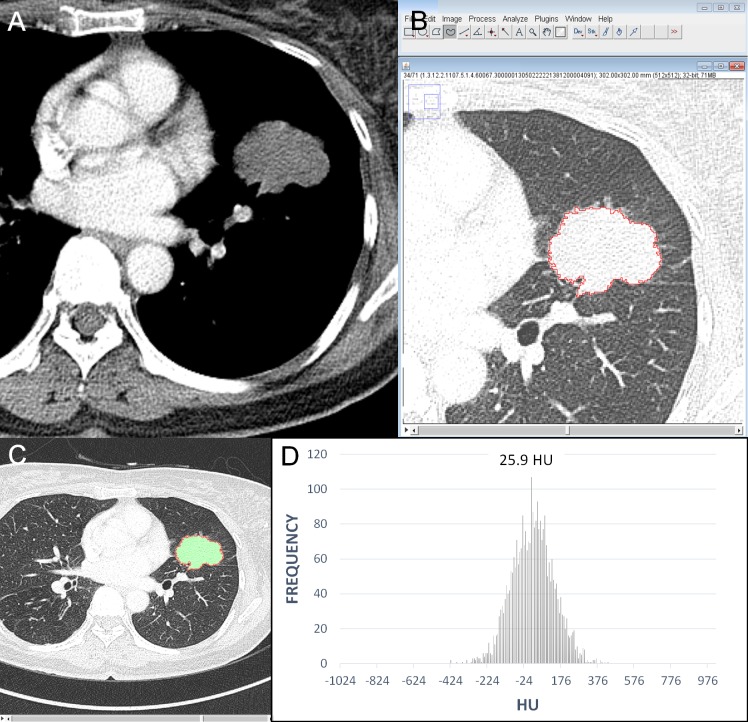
An example of quantitative CT analysis in an adenocarcinoma without recurrence. (A) CT scan and (B) screenshot of quantitative analysis show a 4.6-cm irregular nodule in the left upper lobe (T2aN1M0, stage IIA). (C) The graph shows CT number (Hounsfield unit) distribution of the tumor. The perimeter of the tumor was 159.02 mm; area of tumor was 1020.79 mm^2^; mean attenuation, 25.91 HU (Hounsfield unit); circularity, 0.51; aspect ratio 1.48; roundness, 0.67; skewness, 0.014; kurtosis, 0.21; and entropy, 7.06. The tumor attenuation is low, and is expected to no recurrence and the patient alive without recur for two years.
